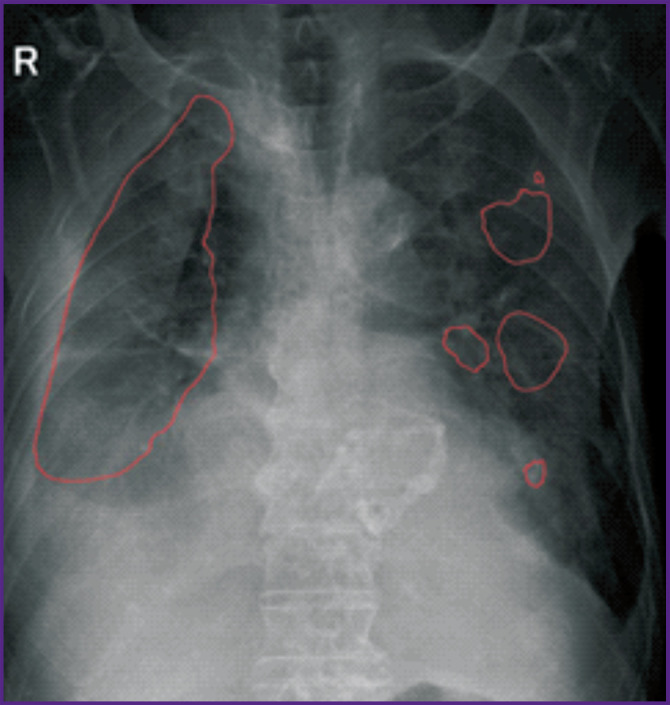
An example of SaMD-AI technical flaws (2.5 and 2.7, see the Table) using evidence from chest radiography
